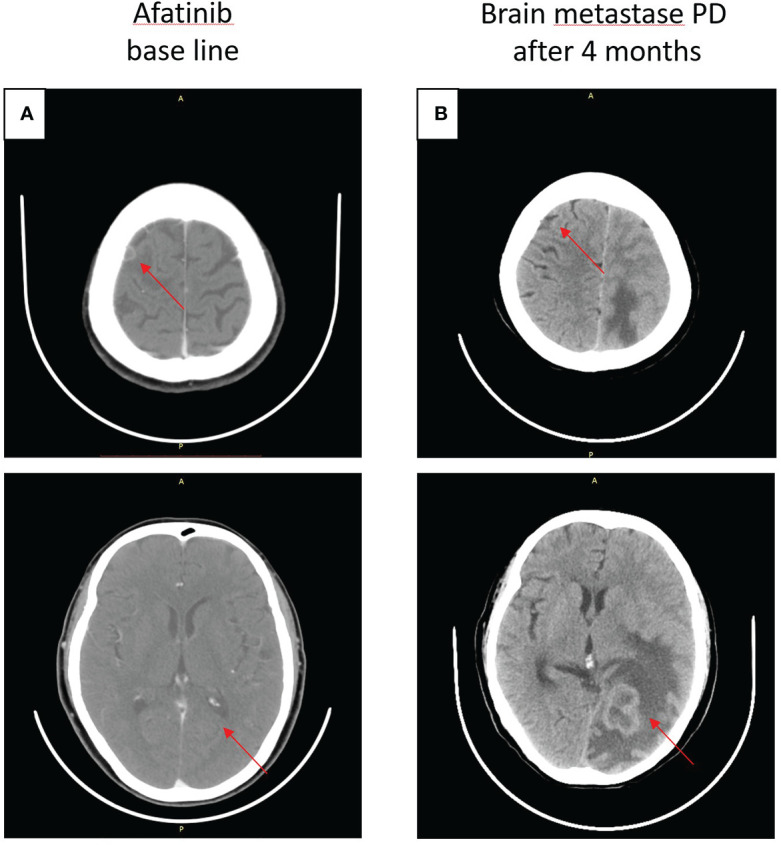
Craniocerebral CT about clinical response to afatinib therapy at different times. (A) Baseline before afatinib treatment. (B) Brain metastases progressive disease (PD) after 4 months.
